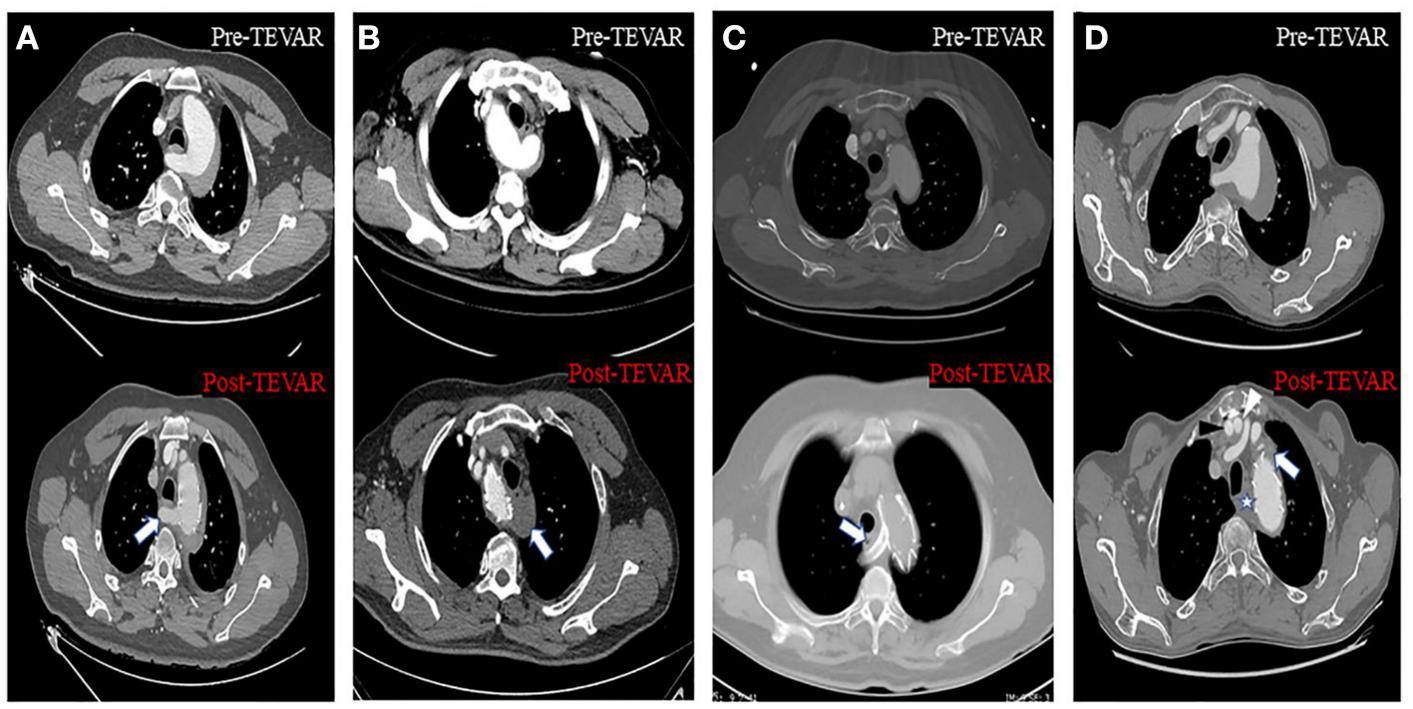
Axial CT images about four different approaches to solve the inadequate proximal landing zone when TEVAR was performed. (A) Handmade fenestrated stent graft: arrow showed that the blood flow of the aberrant right subclavian artery (ARSA) was preserved. (B) Aberrant left subclavian artery (ALSA) was covered without non-revascularization, and arrow showed the thrombosis at the origin of the ALSA. (C) Chimney stent technology: arrow showed the ARSA was patent after the chimney stent was implanted. (D) Hybrid repair: black and white arrowhead showed the artificial blood vessel from aorta to right and left subclavian artery, respectively; star and arrow showed the thrombosis at the origin of the ARSA and left subclavian artery (LSA), respectively.
